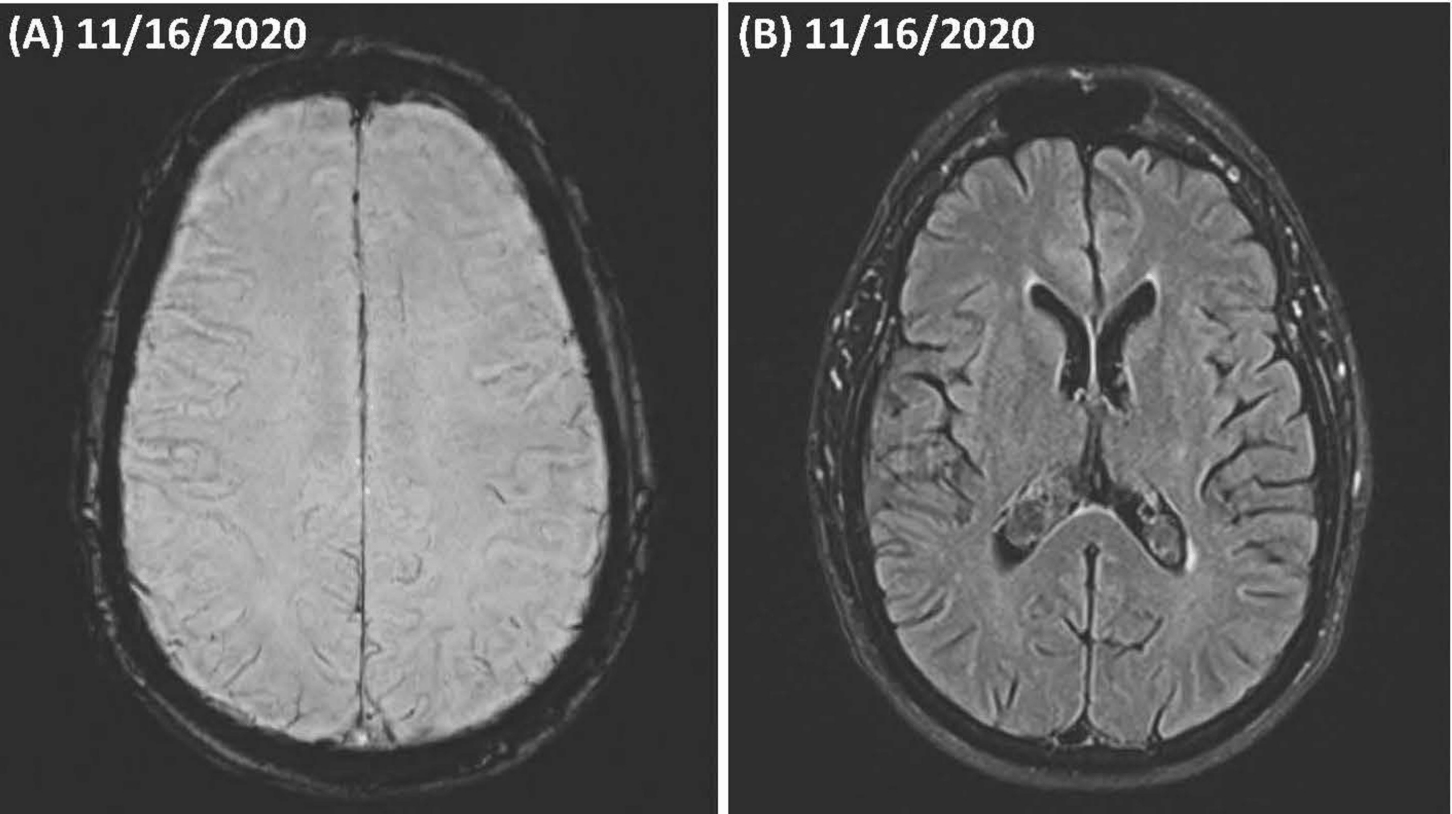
Brain MRI post-concussion (A) Susceptibility-weighted imaging (SWI) sequence showing no hemosiderin deposition. (B) Fluid-attenuated inversion recovery (FLAIR) sequence imaging showing mild hyperintensities of frontal and parietal periventricular white matter.
